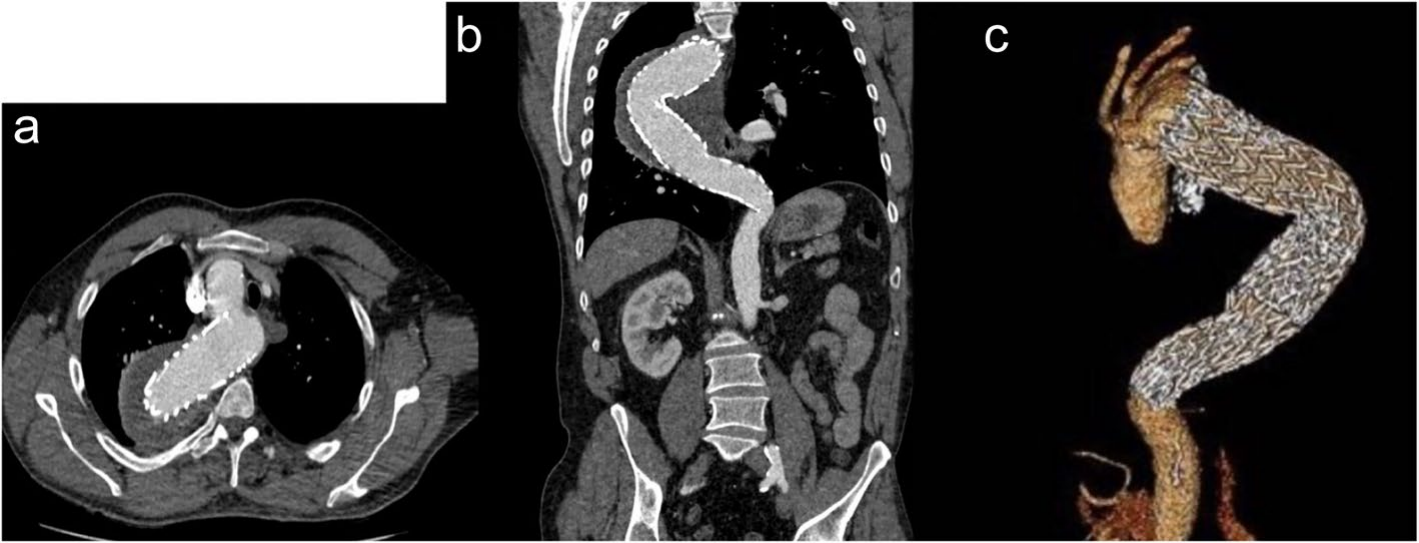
Post- CTA 10 months later: a) axial reconstruction of the stent graft in the right sided aortic arch b) coronal reconstruction of the stent graft in the descending aorta c) volume rendering
